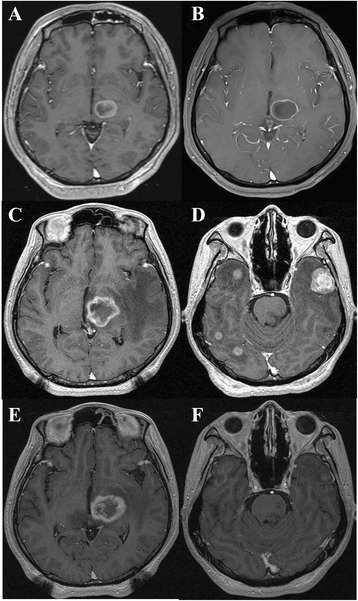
a. A gadolinium(Gd)-enhanced brain magnetic resonance (MR) image depicts a single metastasis (arrow) in the left thalamus. b and c. Follow-up Gd-enhanced brain MR images demonstrate tumor progression (arrow) in the left thalamus. d. A follow-up gadolinium-enhanced brain MR image shows several new metastases (arrows) with surrounding edema in both cerebral hemispheres. e and f. Follow-up Gd-enhanced brain MR images after anti-PD-1 therapy demonstrates the stable state of the repeatedly treated thalamic metastasis (arrow) and nearly complete resolution of the newly developed metastases
